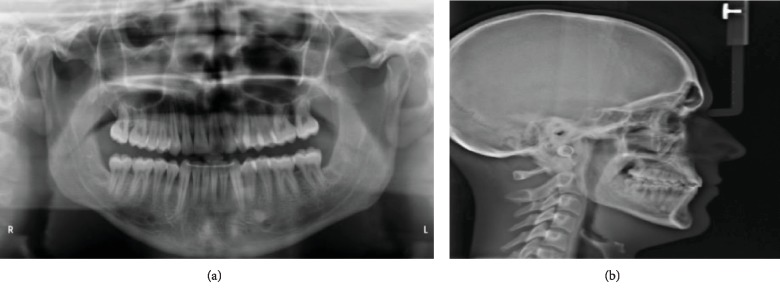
(a) Posttreatment panorama showing levelling of the upper and lower left posterior segments. (b) Posttreatment cephalometric X-ray showing minor skeletal changes with slight changes in the upper and lower incisor inclination.
